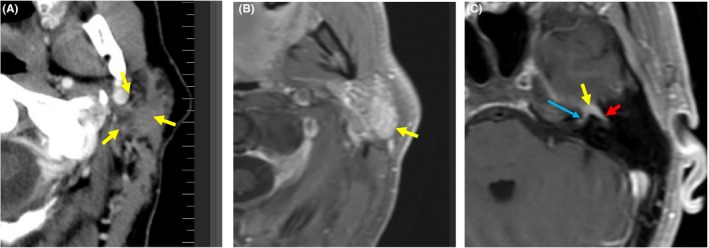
Pretreatment imaging. A, Axial computed tomography image status post contrast administration demonstrating nonenhancing mass in the left parotid gland (arrows). B, Axial T1‐weighed fat‐suppressed postgadolinium MR image demonstrates enhancing mass (arrow). C, Axial postcontrast fat‐suppressed T1‐weighted MR image demonstrating tumor involvement of the geniculate ganglion (yellow arrow), labyrinthine (blue arrow), and tympanic (red arrow) segments of the left facial nerve
